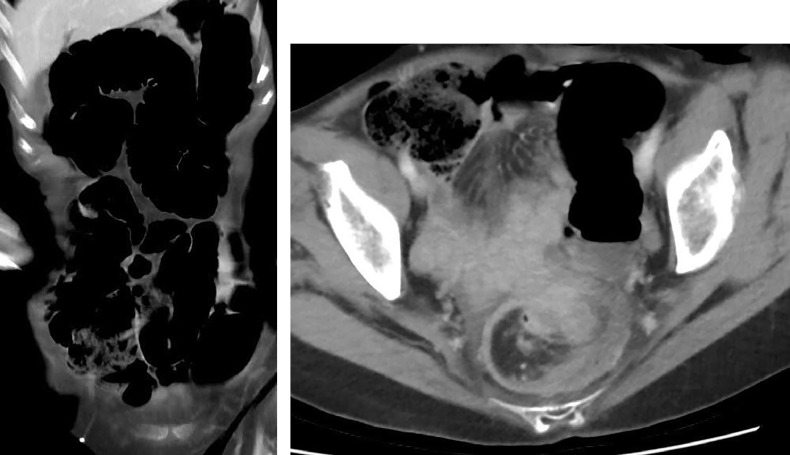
CT scan with coronal and axial views and thick cuts showing large bowel dilation.
